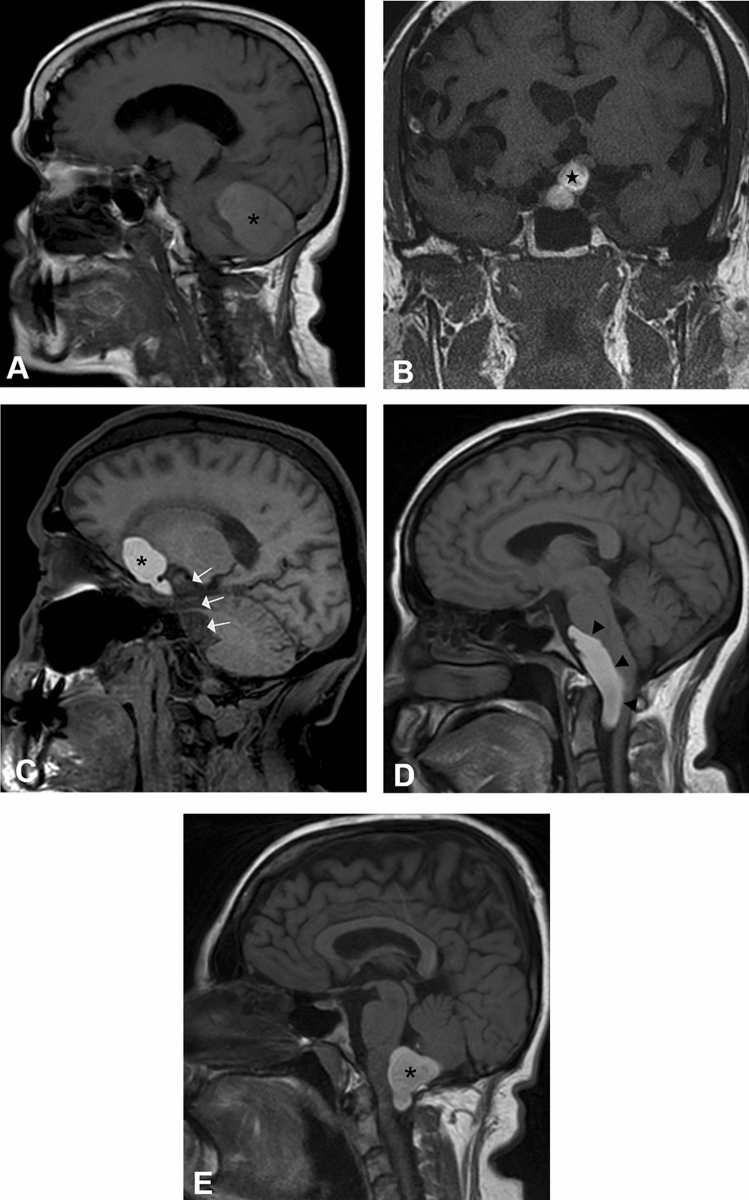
Non-enhanced T1-weighted MR images of the brain demonstrate spontaneously hyperintense white epidermoid cysts. Patients 1, 4 and 5 (A,D,E) have lesions in the posterior fossa, with extension to spinal canal in patients 4 (black arrowheads) and 5 (black asterisk). Patient 2 (B) presents with a sellar/suprasellar lesion (black star). The lesion in patient 3 (C) has 2 components: classic epidermoid posteriorly (white arrows) and white epidermoid anteriorly (black asterisk).
